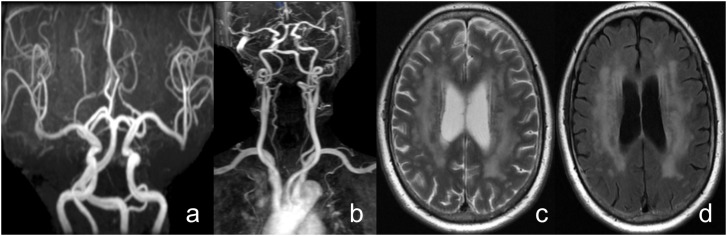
A female patient, 71 years old, with complaints of “vertigo, nausea” and a history of hypertension. (a) Head MRA shows vertebrobasilar artery dolichoectasia, (b) Neck contrast enhanced MRA shows right vertebral artery dysplasia and bilateral vertebral artery tortuosity, (c,d) T2WI and FLAIR shows the side body of the lateral ventricle white matter high signal, Fazekas grade 3.
